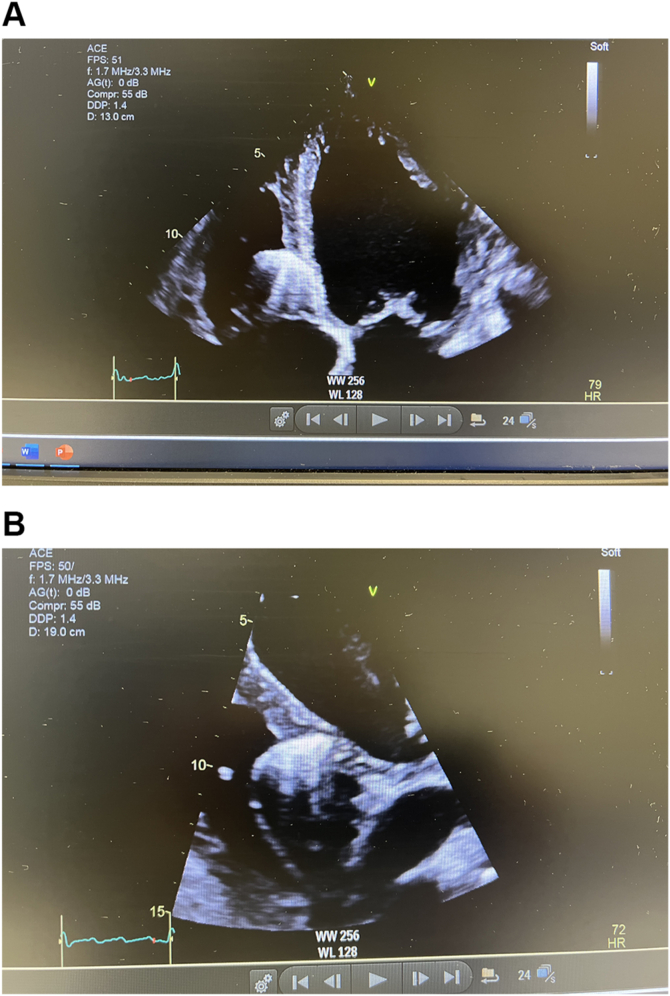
A: Apical 4-chamber view 8 months after permanent pacemaker implant showing stable ventricular-lead position in the left subendocardium. B: Modified parasternal view 8 months after implant showing a stable ventricular-lead position in the left subendocardium.
